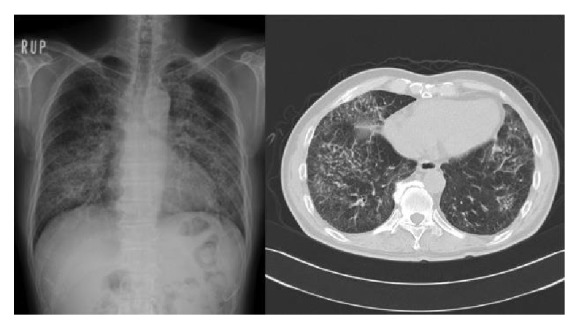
Chest radiography and CT findings at the first visit. Diffuse fine nodular or reticular shadow in both lung fields (predominant in lower lungs) was seen.
